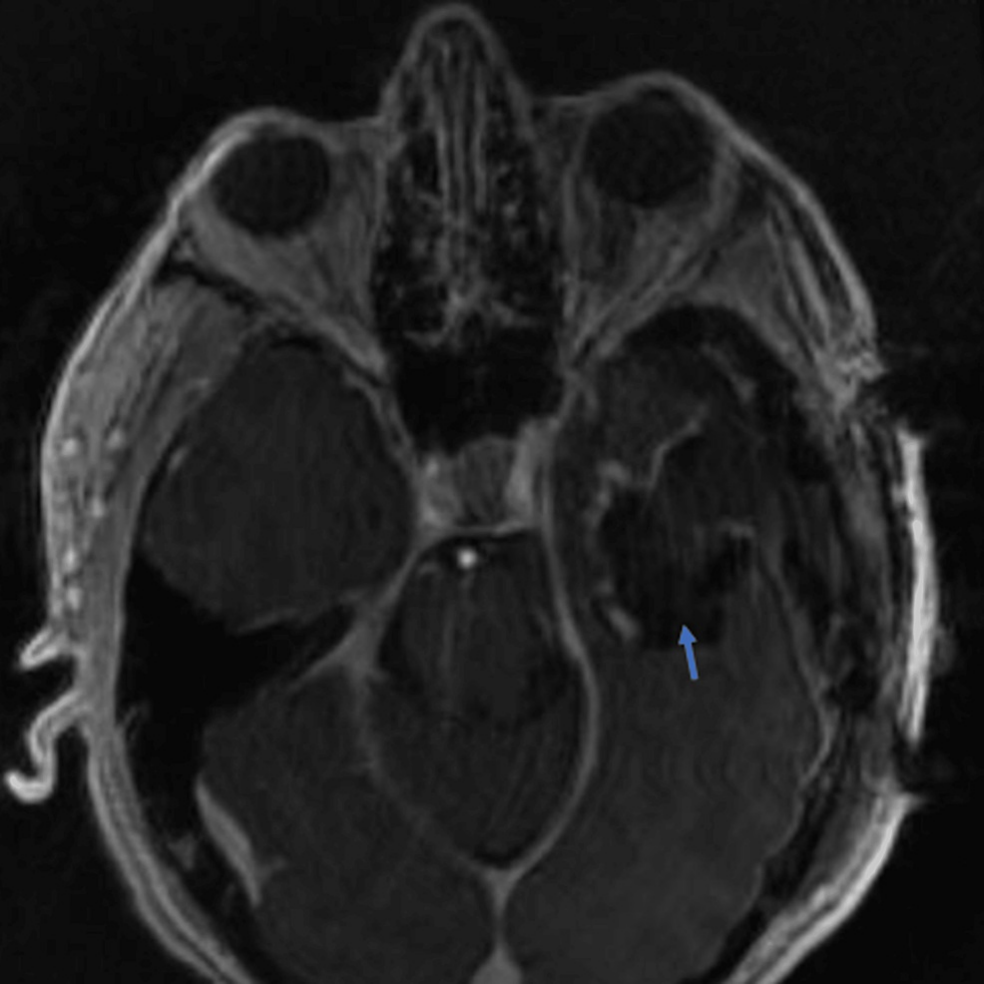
Postoperative MRI: axial T1 with contrast The view shows the gross total resection of the lesion.
